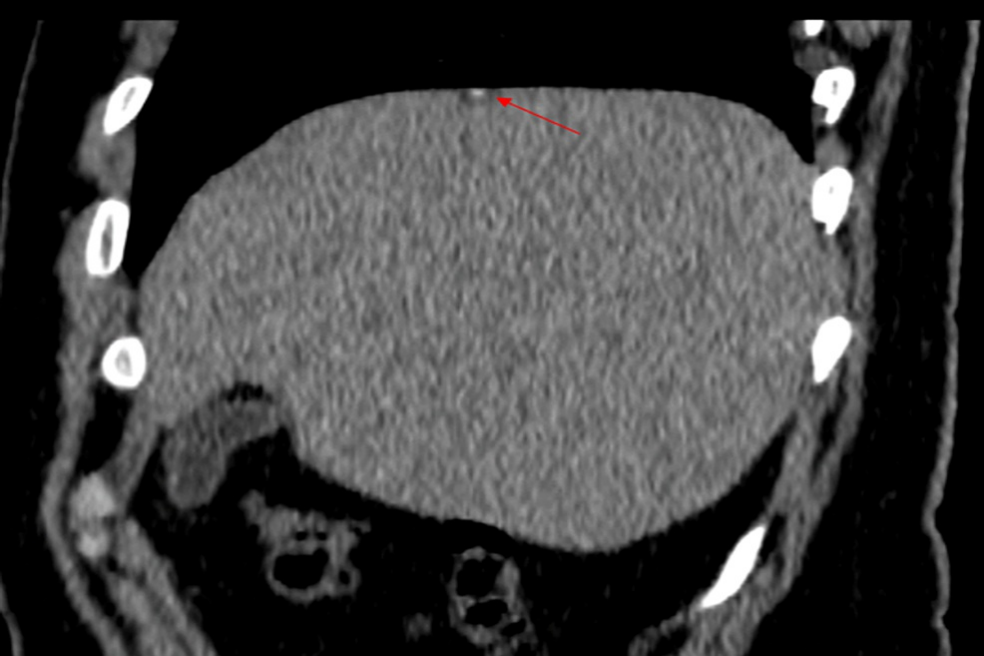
Sagittal CT: cystic lesions within the liver dome with a fleck of calcification.
